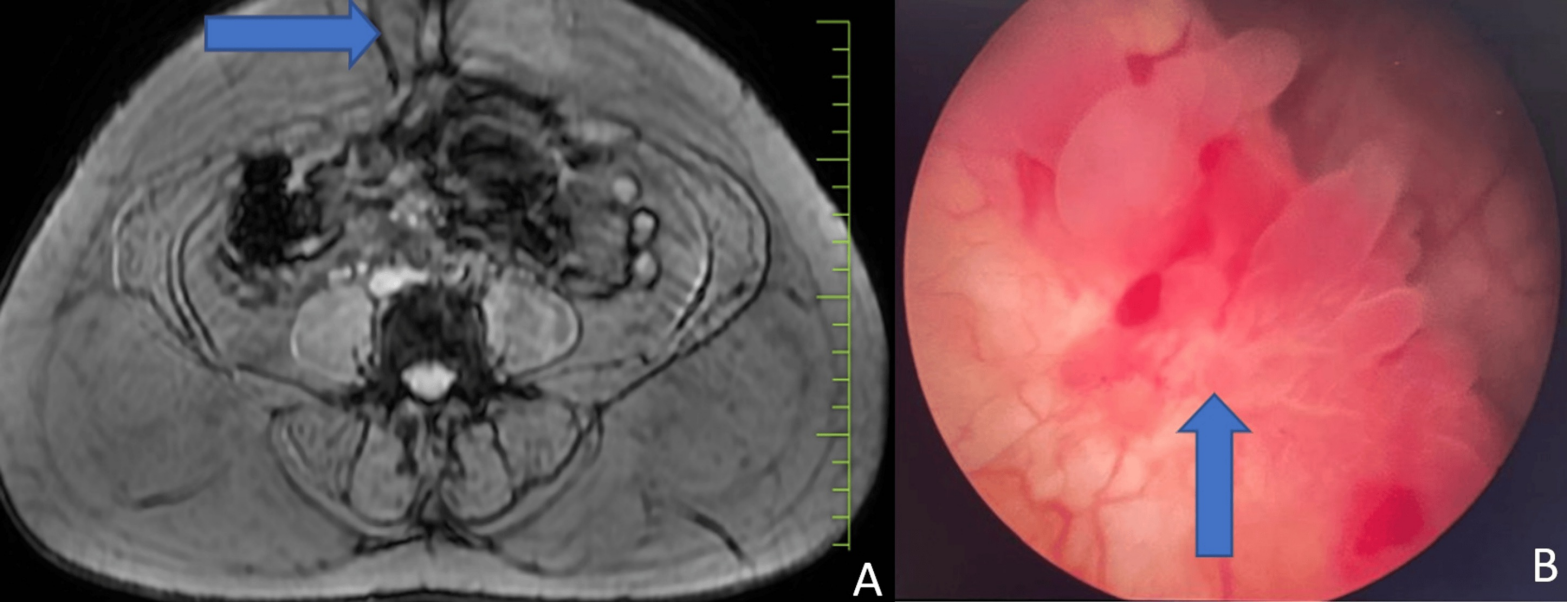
(A) MRI scan of the abdomen and pelvis, showing a T1 hyperintense lesion in the umbilical region, with a blue arrow pointing to the umbilical nodule. (B) Cystoscopy showing a 2 x 2 cm polypoidal lesion in the posterior wall of the bladder, with a blue arrow pointing to the lesion in the bladder.
